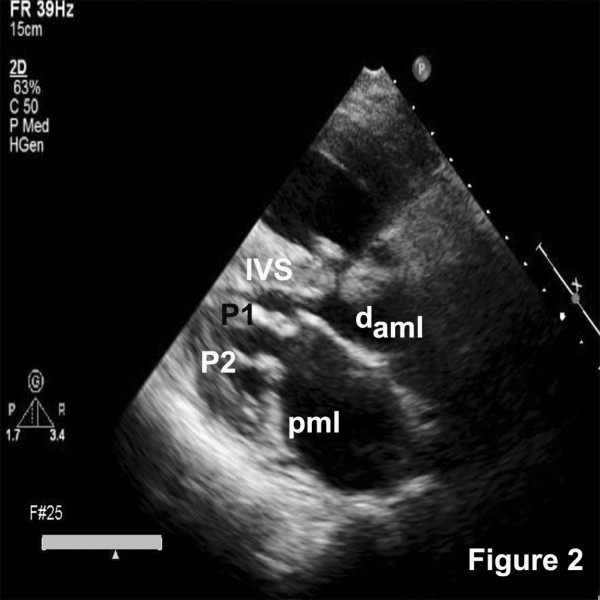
Parasternal long axis view demonstrating the papillary muscles (1 and 2), doming of the anterior mitral leaflet (d_aml_) and fixed posterior mitral leaflet (pml); IVS, interventricular septum; LA, left atrium.
